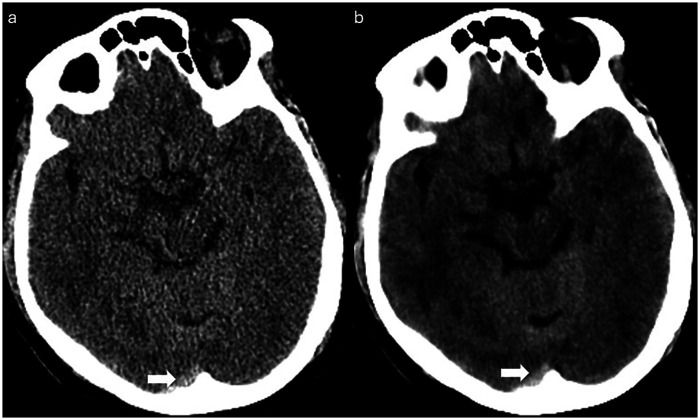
Axial CT LD images of a 56-year-old woman with right occipital acute extradural hemorrhage. The small hyperattenuation area was clearly delineated in DLIR (b) while preserving image noise compared with iDose^4^
